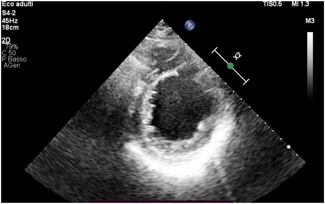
Ventricular septal defect seen with transthoracic echocardiography
